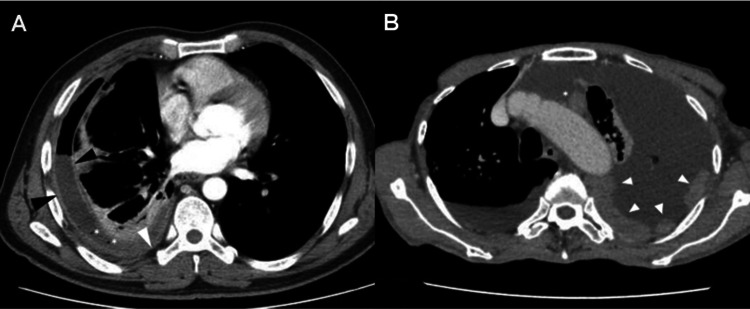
Some CT morphological features of pleural effusion. A A 51-year-old male, benign pleural effusion (BPE) with pleural contrast enhancement (black arrowhead), air bubbles in pleural space (white asterisk), and increased attenuation of the extrapleural fat (white arrowhead). B A 79-year-old female, malignant pleural effusion (MPE) with diffuse pleural thickening (white arrowhead) and enlarged mediastinal lymph node (white asterisk)
